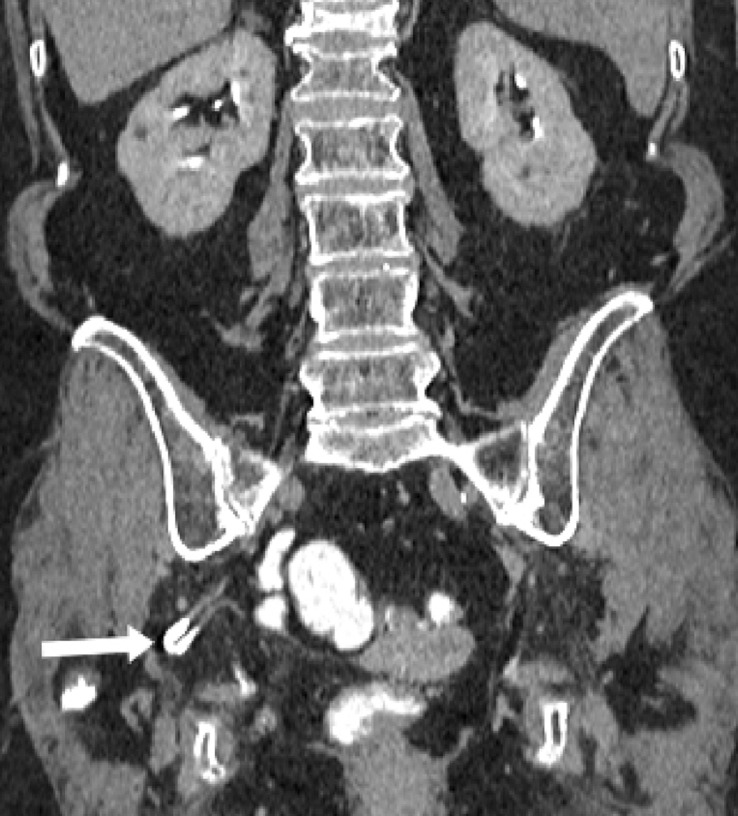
Coronal CT image in the delayed phase demonstrates a right ureterosciatic hernia with the curlicue sign (white arrow). The ureter is opacified with contrast in this image.
